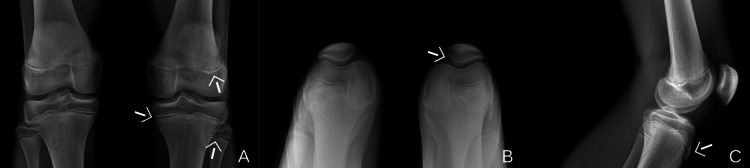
(A) X-ray AP, (B) sunrise view of the knees bilaterally, and (C) lateral view of the left knee. White arrows indicate: (A) open distal femoral and proximal tibia/fibula growth plates; (B) trace lateral displacement of the patella; and (C) elevation of the tibial tuberosity. There are no acute fractures.
